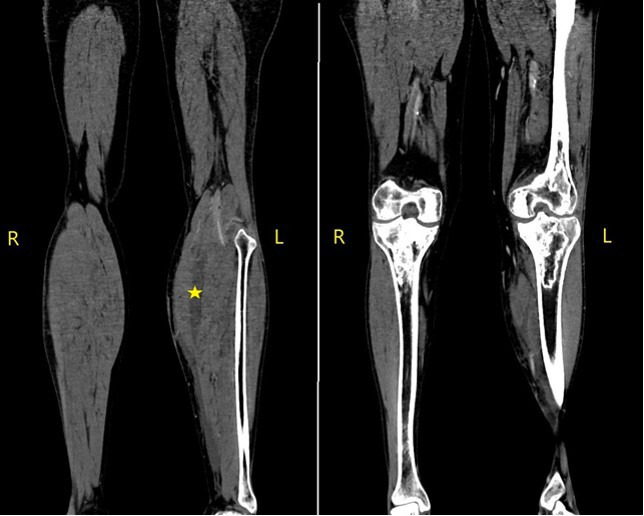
Lower extremity CT scan with intravenous contrast. Signs of inflammatory arthritis with collections that extend primarily between the medial calf and soleus in the left leg. Deep venous thrombosis is not demonstrated. There is no evidence of osteomyelitis despite the findings of bone infarction in both knees.
